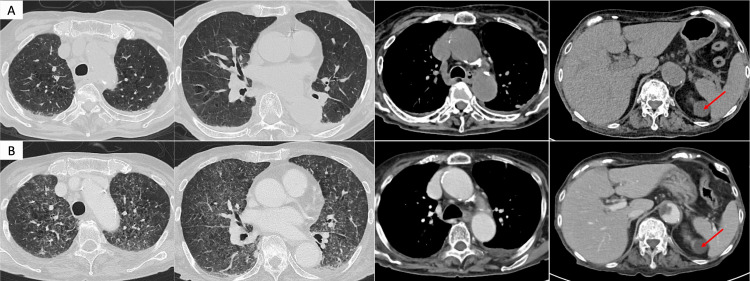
CT findings during hospitalization. (A, B) Non-contrast CT on day 0 (admission) and contrast-enhanced CT on day 13, respectively. (A) There were no abnormal findings in the lung parenchyma except passive atelectasis in the dependent areas. No hepatomegaly or splenomegaly is observed. A round lesion adjacent to the spleen is identified as a left-sided renal cyst (arrow). (B) Widespread, poorly defined small nodules and ground-glass opacities are observed diffusely in both lungs. The liver and spleen exhibited no morphological abnormalities or enlargements. A left renal cyst is observed (arrow). CT: computed tomography
